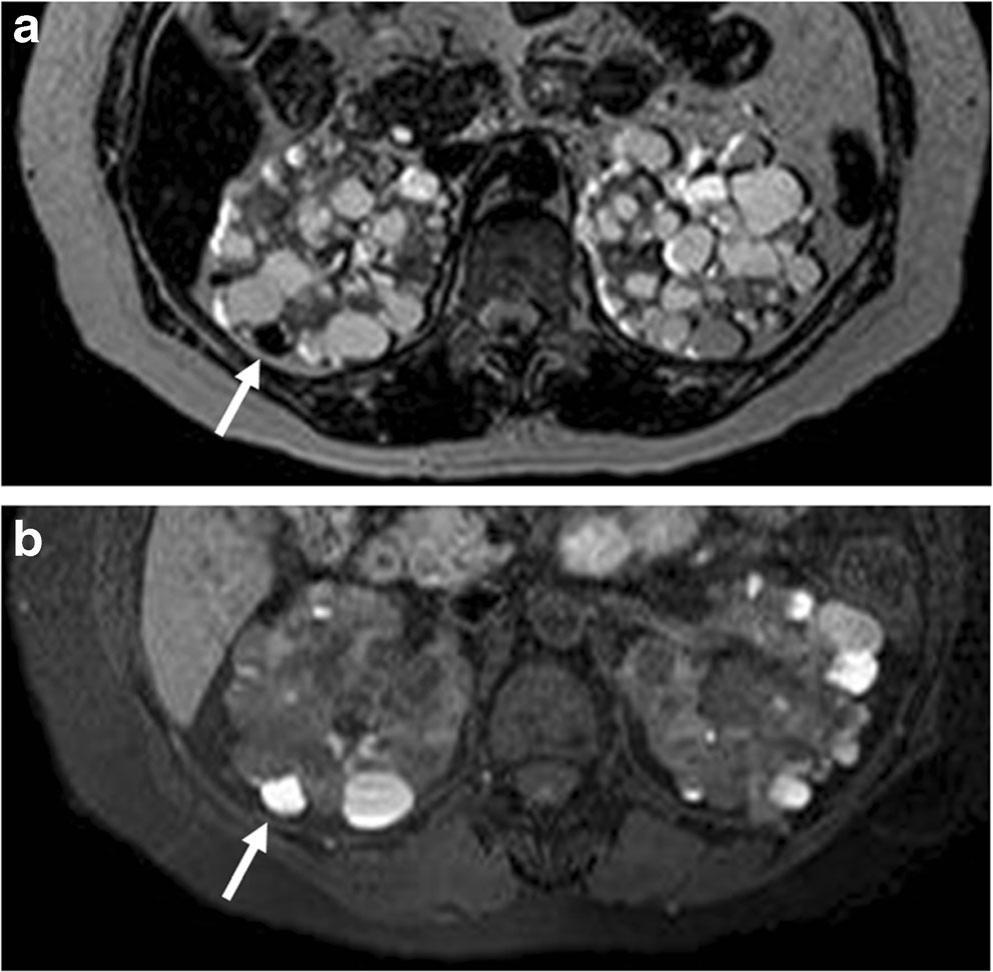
a, b: Haemorrhagic renal cyst (*arrow*) in the right kidney in a patient undergoing long-term haemodialysis. The cyst appears hypointense on T2-weighted MR sequences (a) and hyperintense on T1-weighted MR sequences (b)
